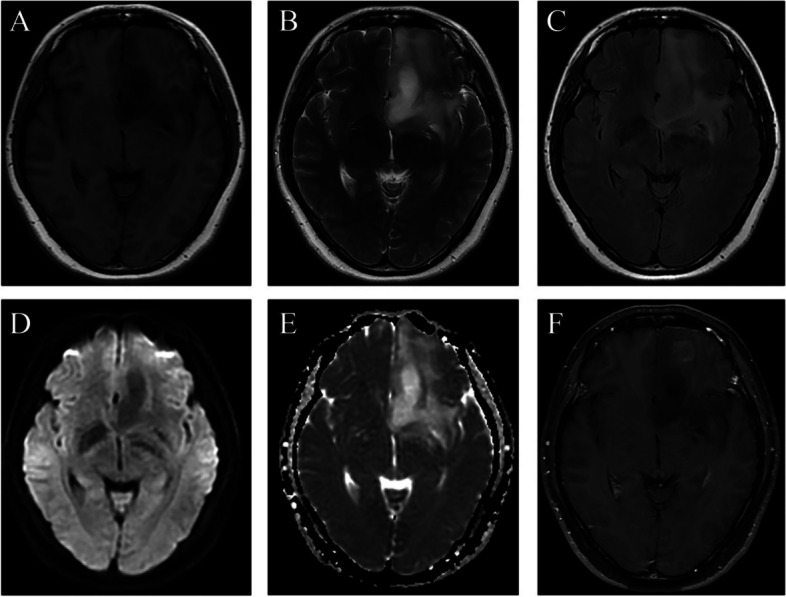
36-year-old female patient with type B APS glioma. The lesion involves the left temporal pole, orbital gyrus, straight gyrus, paraolfactory gyrus, and paraterminal gyrus. It is low signal on T1WI (A), high signal on T2WI (B) and fluid attenuated inversion recovery (FLAIR) (C), low signal on DWI (D) and high signal on apparent diffusion coefficient (ADC) (E). On post-contrast axial image, it demonstrates patchy enhancement (F)
